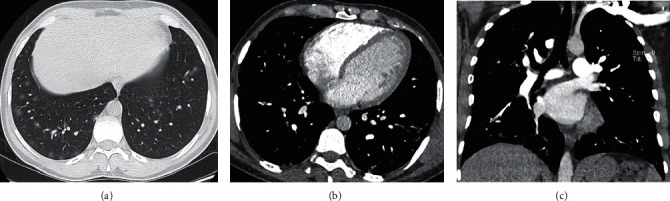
Images in a 38-year-old male with COVID-19 pneumonia complaining of fever and cough. (a) Axial unenhanced chest CT scan (lung window) obtained on day 5 after the onset of symptoms shows bilateral multiple patchy areas of ground-glass opacities more peripherally. (b) Axial CT pulmonary angiography scan. (c) Coronal reformatted images (mediastinum window) on day 19 after the onset of symptoms demonstrate filling defects (white arrow) within the segmental pulmonary artery branch supplying the lateral basal segment of the right lower lobe.
